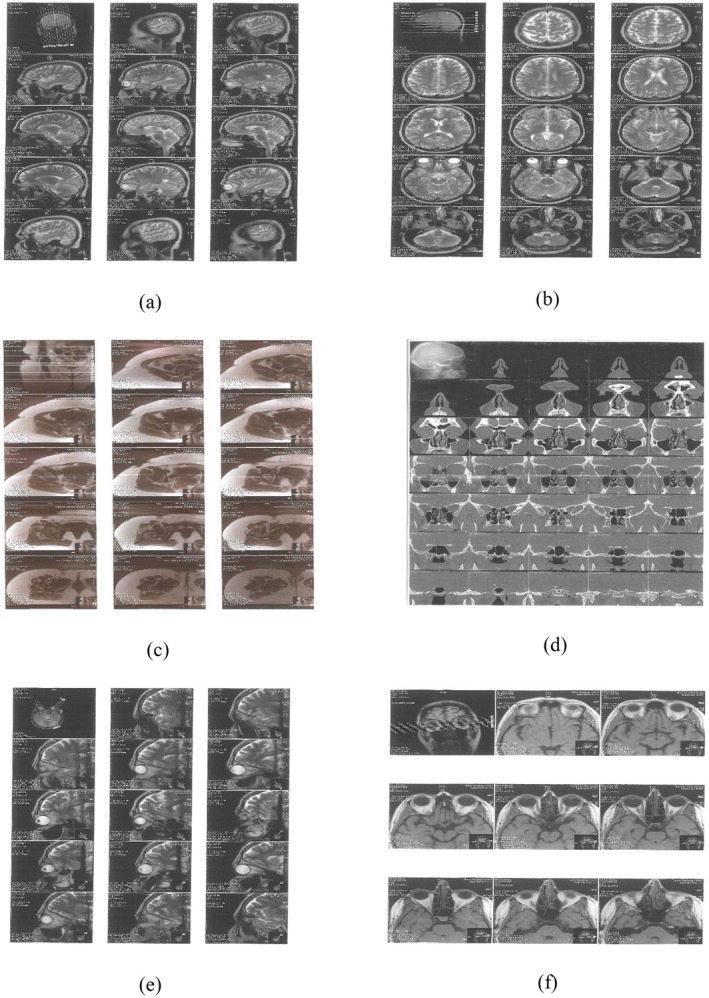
Normal diagnostic imaging findings prior to tDCS treatment (March–April 2024). (a, b) Contrast‐enhanced axial T1‐weighted brain MRI. (c, d) Orbital CT scans. (e, f) Nasal endoscopy views. No abnormalities were detected.
